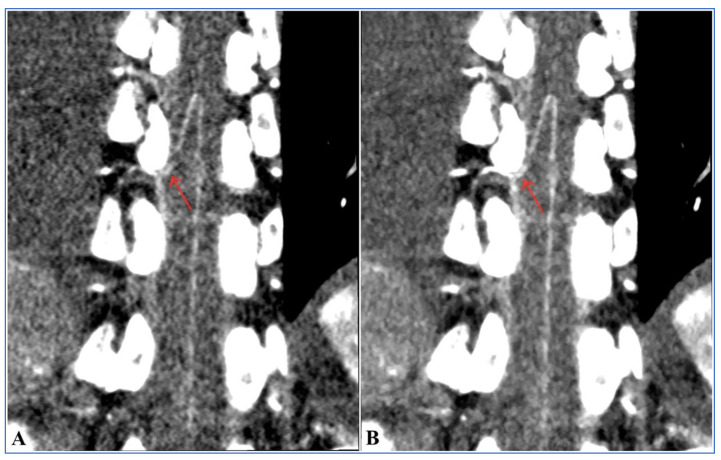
Preoperative 100 kV CTA images of a 55-year-old female patient with aortic aneurysm obtained with a small field of view. (A) FBP reconstruction showed high image noise and unsatisfactory visualization of the AKA (red arrow), especially at the intervertebral foramen, with a subjective image quality score of 3 and a visualization score of 3. (B) ASIR-V 80% reconstruction showed obviously reduced image noise and significant improvement in visualization of the AKA artery (red arrow) which can also determine the level of origin of the AKA with a subjective image quality score of 5 and visualization score of 5. CTA = computed tomographic angiography; ASIR-V = adaptive statistical iterative reconstruction-V; AKA = Adamkiewicz artery; and FBP = filtered back projection.
